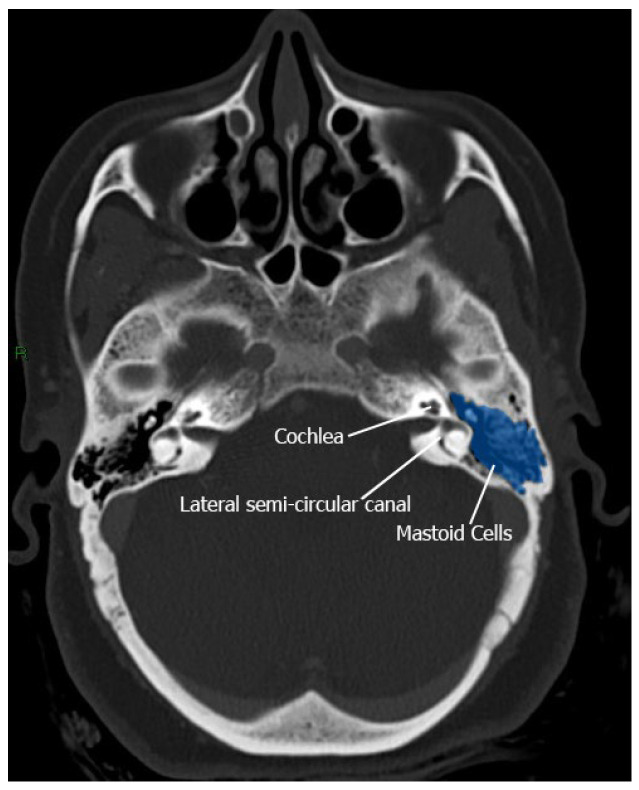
Mastoid air cells and their relation to vestibular structures. An axial CT image at the level of the temporal bone shows the mastoid air cell system (highlighted in blue on the left side). The labeled key anatomical landmarks include the cochlea and lateral semicircular canal, demonstrating the close proximity (2–8 mm) between the pneumatized mastoid and the vestibular labyrinth, which contains temperature-sensitive endolymph. The CT image shown is a de-identified institutional scan used retrospectively with institutional ethics approval. The cochlea (approximately 7–9 mm in diameter) provides anatomical scale reference.
